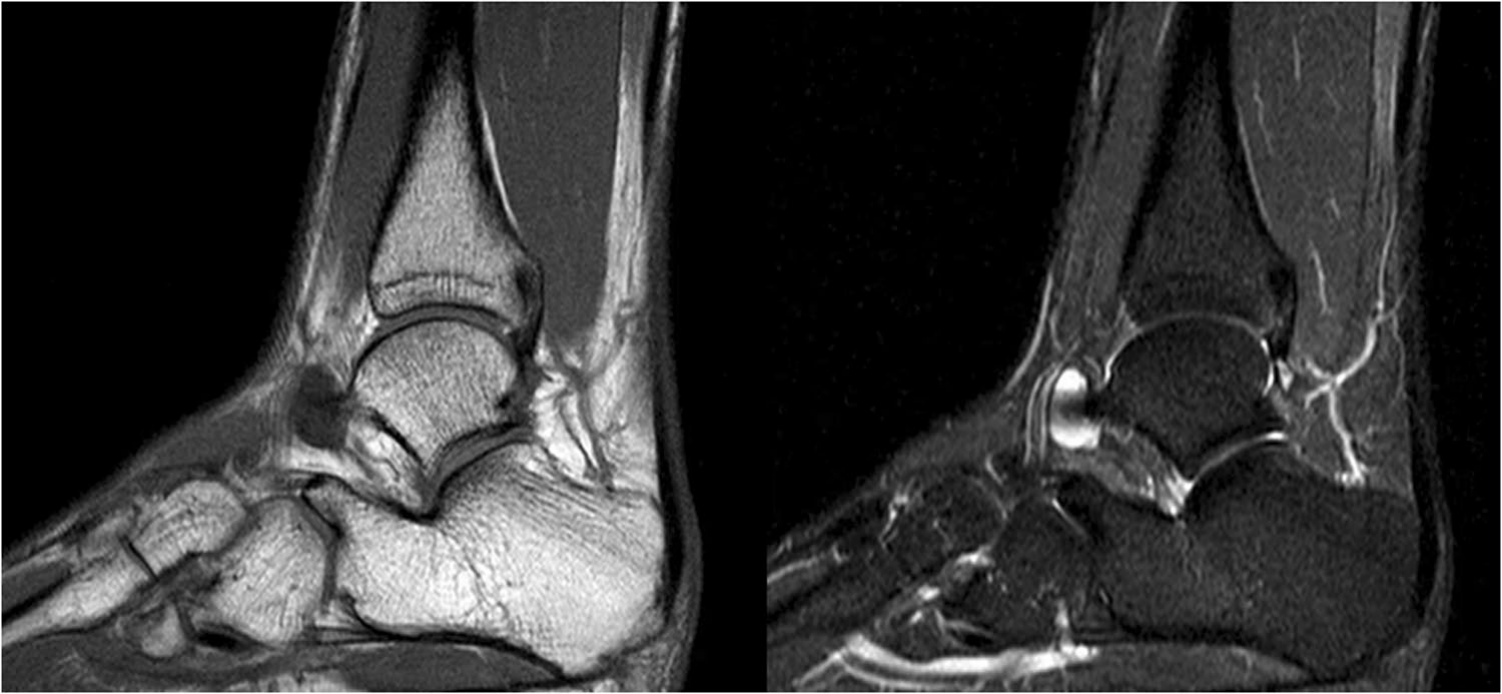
Sagittal MRI Coupes of a ganglion cyst. A ganglion cyst of 1.7 × 1.1 × 0.6 cm on the anterolateral side of the talar neck originating from the sinus tarsi (Left: T-1 Weighted, Right: Short-TI Inversion Recovery (STIR))
